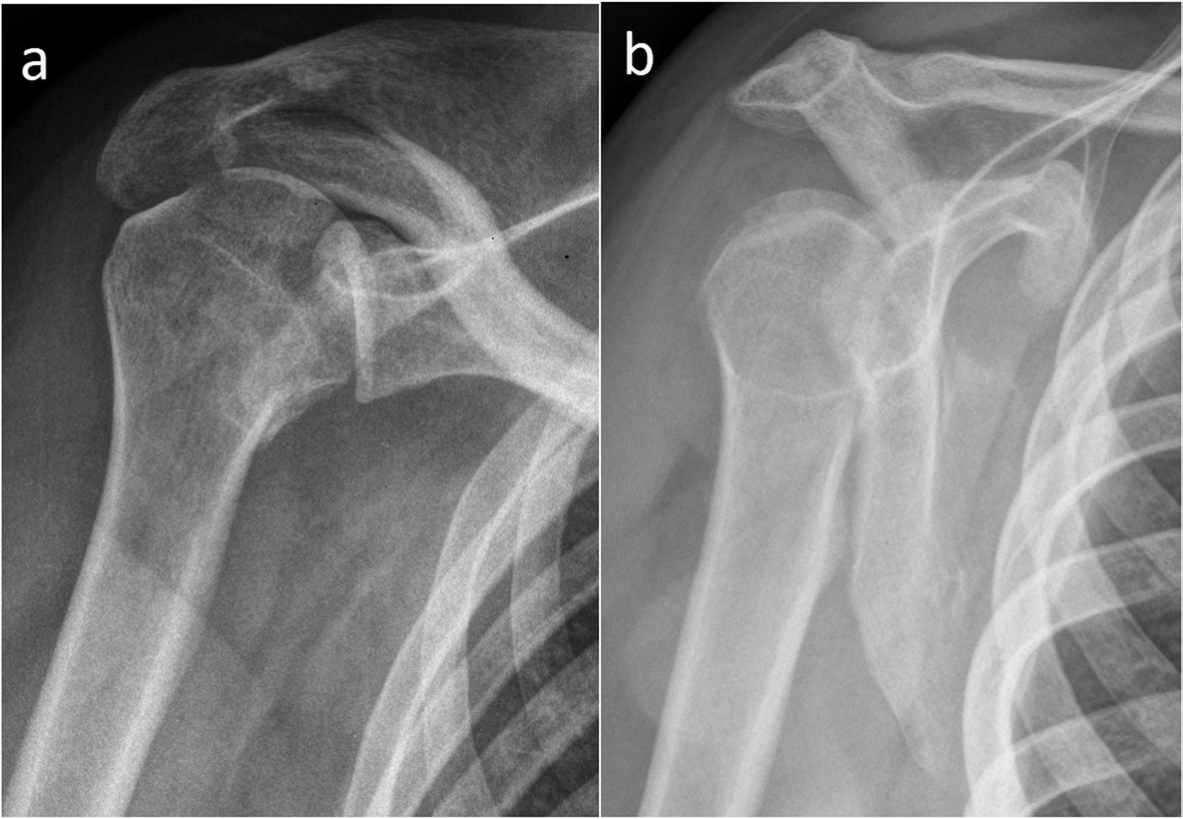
Locked posterior shoulder dislocation (right shoulder), preoperative imaging. a Anteroposterior and (b) scapular Y view X-ray images of the right shoulder of a patient with locked posterior shoulder dislocation
